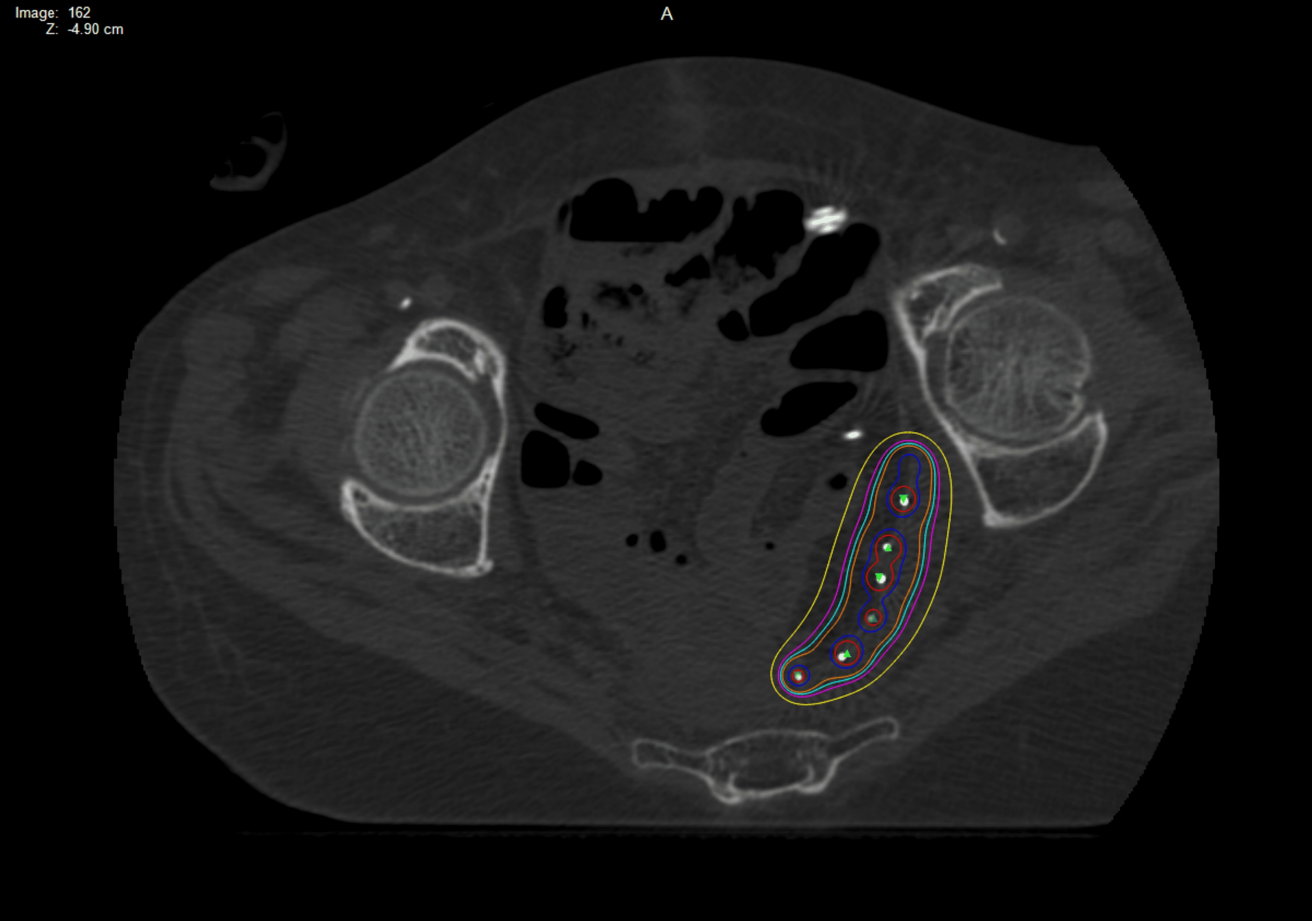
Post-implant CT scan with the Vicryl mesh with Cesium-131 stranded seeds in the expected location for case 2 Red = 200% isodose line (ISL); blue = 150% ISL; orange = 100% ISL; teal = 90% ISL; magenta = 80% ISL; yellow = 60% ISL
